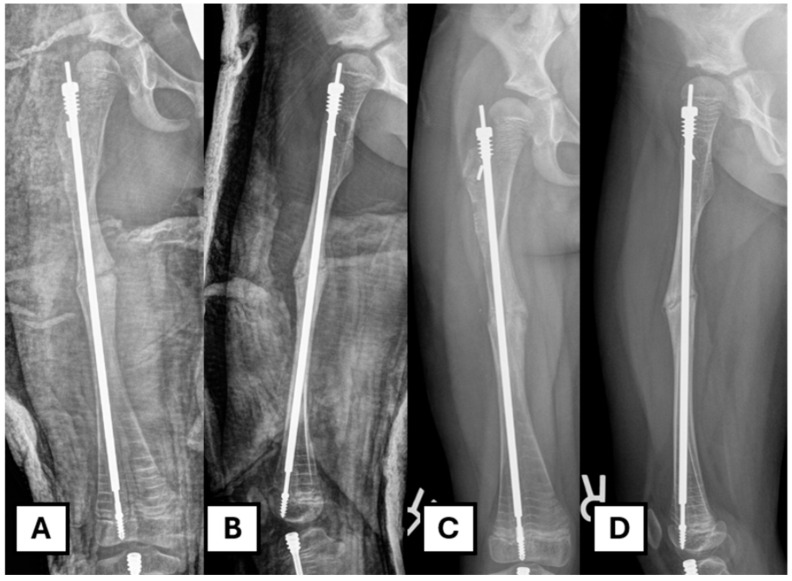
Post-operative image after revision. (A,B) Immediate post-operative radiograph. (C,D) Six weeks post-operative radiograph showing progression of osteotomy site healing.
